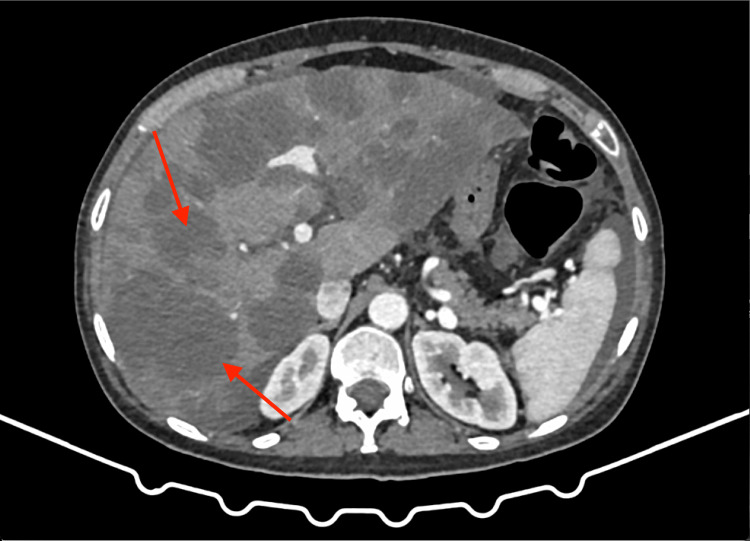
CT of the abdomen showing multiple liver masses concerning for metastases (red arrows)
